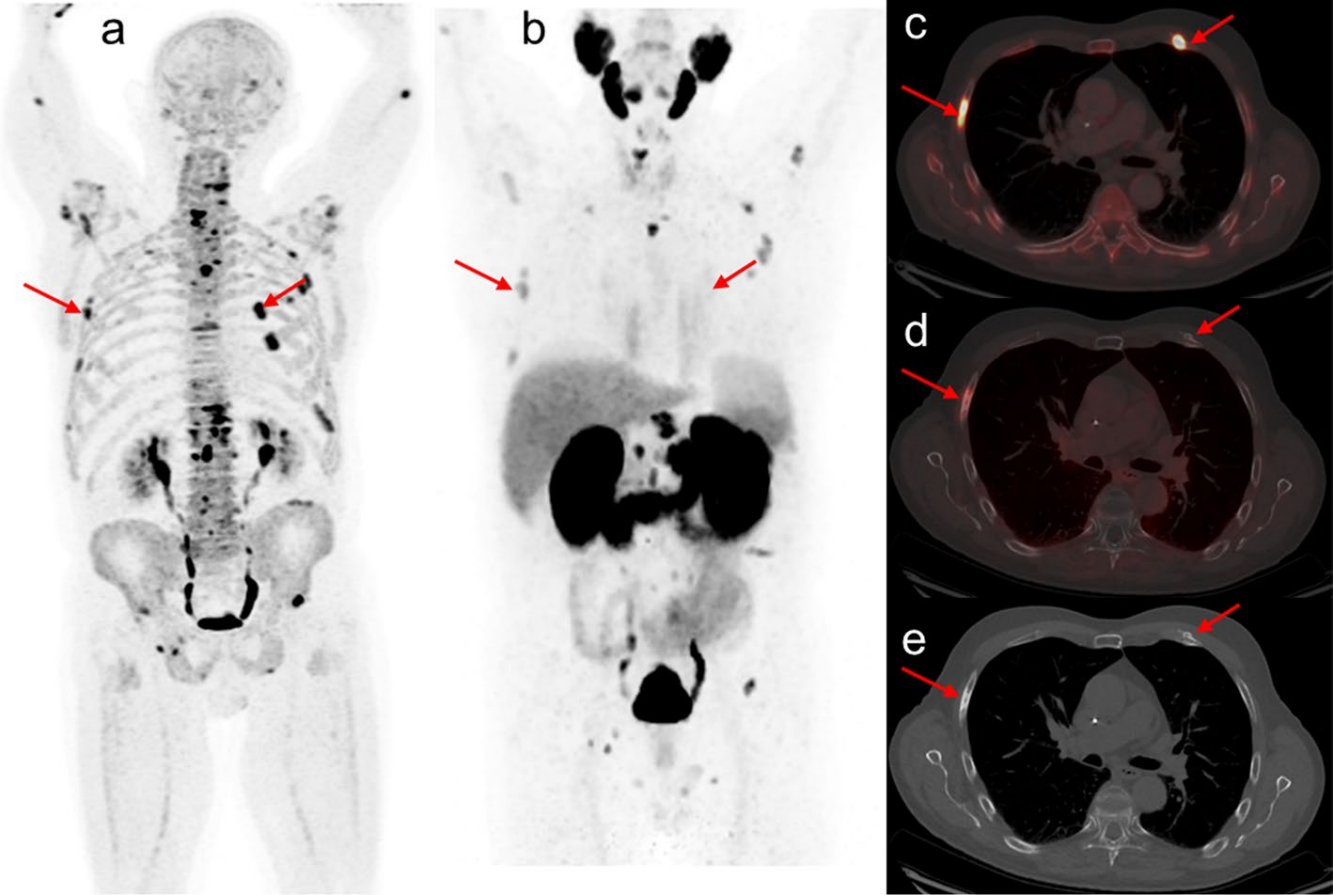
A 65-year-old patient diagnosed with 4 + 3 PCa underwent radiation, hormonal and chemotherapy and became hormone refractive. On referral, PET imaging revealed bone lesions on maximum intensity projection of fluoride PET (a) and PSMA PET (b). The majority of bone lesions were sclerotic in nature having high intensity uptake on fluoride PET (a) but reduced uptake on PSMA PET (b). Fused transaxial PET/CT images showing two sclerotic rib lesions on fluoride PET (c), whereas PSMA PET showed only one sclerotic lesion (d, left arrow over rib). Respective sclerotic lesions showed on axial CT (e)
